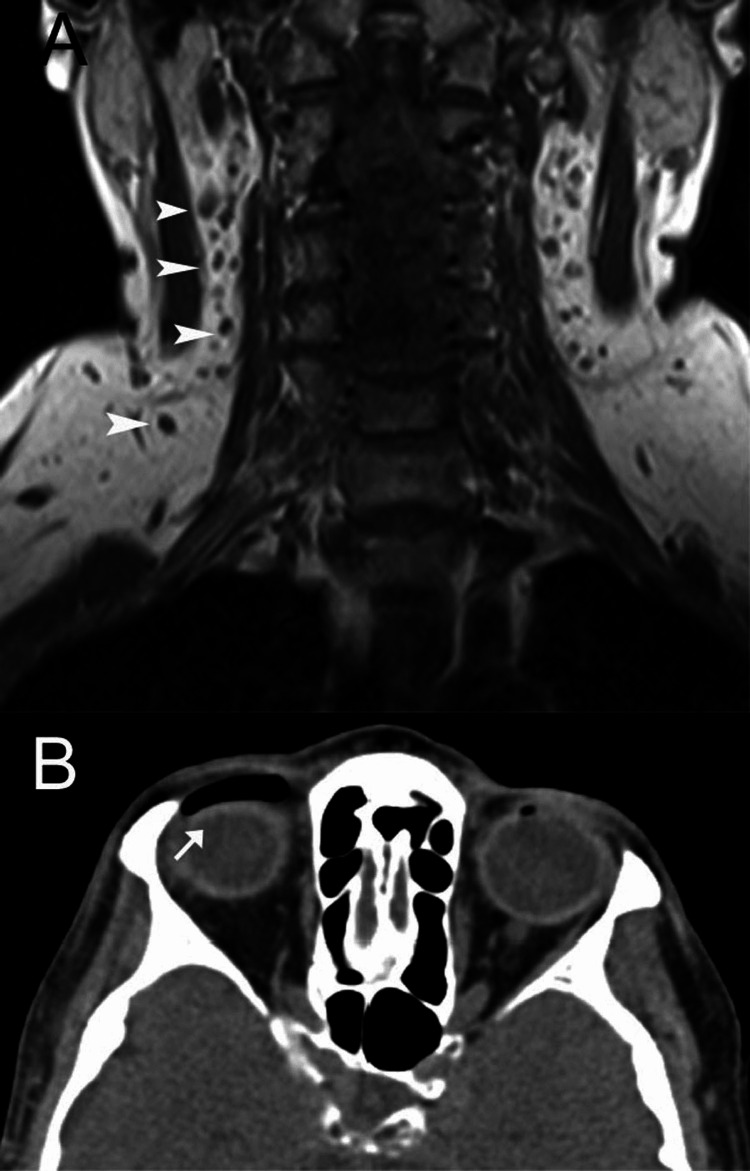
Scans of a 72-year-old woman who developed craniocervical pneumatosis after a tooth extraction A: The coronal T1-weighted MR image shows numerous air bubbles (arrowheads) in the neck; B: On the CT image, a pocket of air is seen in the upper lid (arrow).
